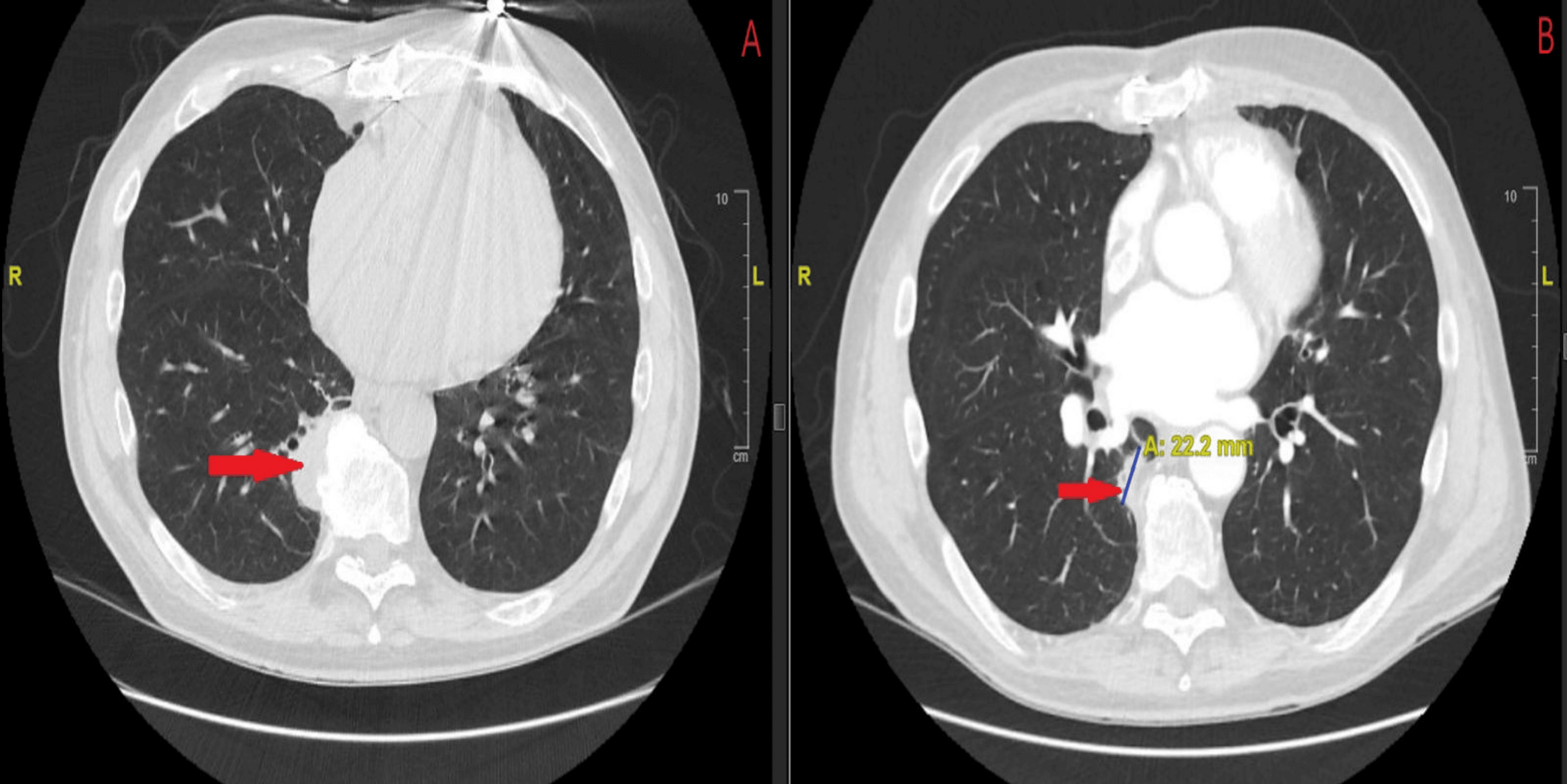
Axial contrast-enhanced CT of the chest demonstrating a pleural-based right lower lobe mass with irregular margins and partial cavitation (arrows). The lesion abuts the posterior pleura and spinous process and corresponded to a hypermetabolic focus on PET imaging. CT: computed tomography; PET: positron emission tomography
